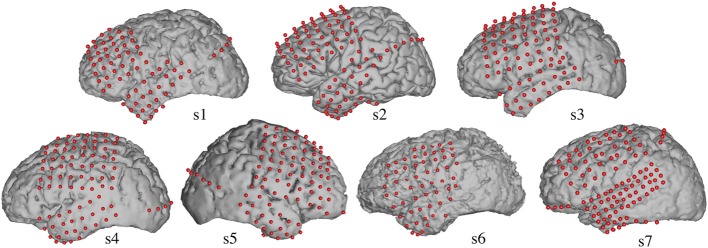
Electrode locations. Grid locations for each subject are overlaid on cortical surface reconstructions of each subject's MRI scan.
